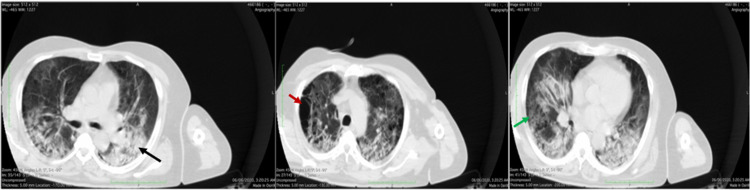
CT chest on day 1 showing baseline emphysematous changes (red arrow) and ground-glass opacities (green arrow) with consolidative changes (black arrow) involving both lungs, mainly dependent areas.
